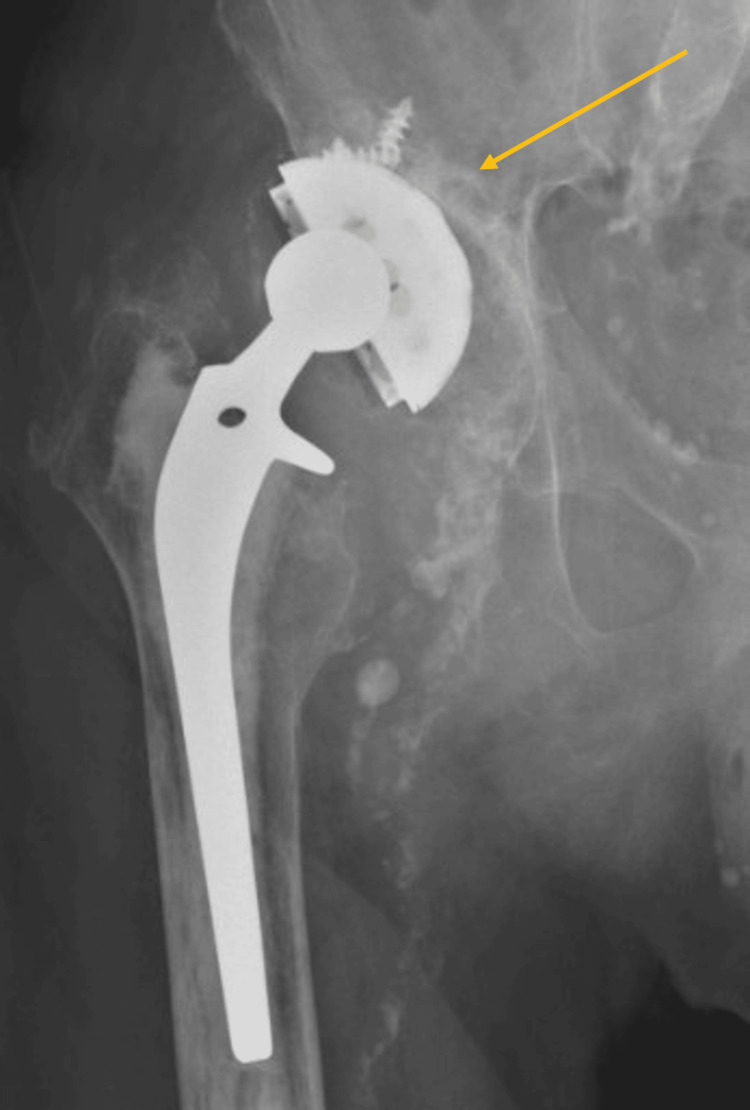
Anteroposterior pelvic X-ray of an 88-year-old patient with aseptic loosening and migration of the acetabular component (grade IIIB Paprosky acetabular defect).
